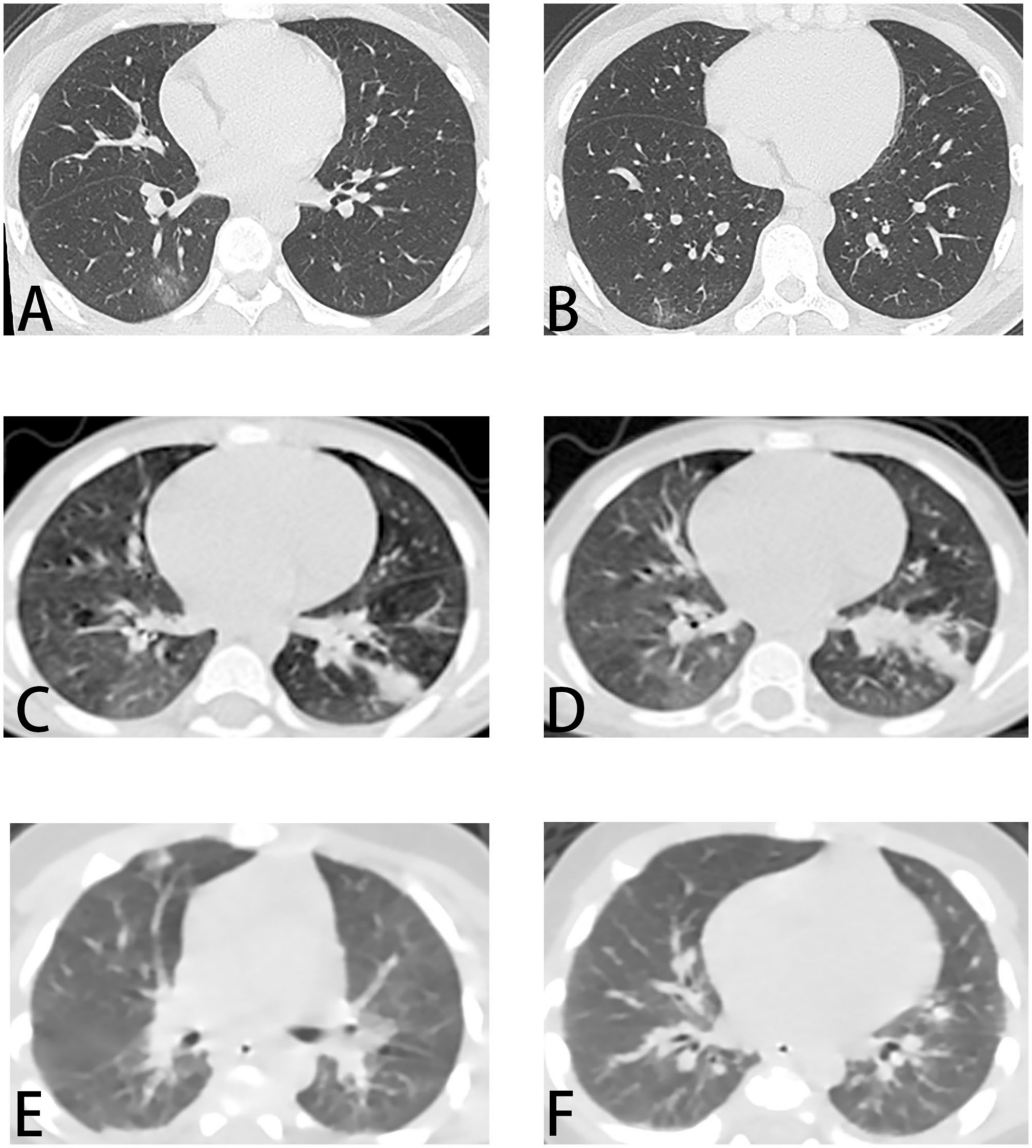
Chest CT images of patients. (A,B) COVID-19. Male, 15 years old. On admission, chest CT showed ground-glass opacities in the inferior lobe of the right lung. (C,D) Mycoplasma pneumonia. Male, 4 years old. Chest CT showed bilateral lesions with consolidation in inferior lobe of the left lung. (E,F) Other viral pneumonia (respiratory syncytial virus). Male, 1 month old. Chest CT showed diffused consolidations in both lungs. CT, computed tomography.
